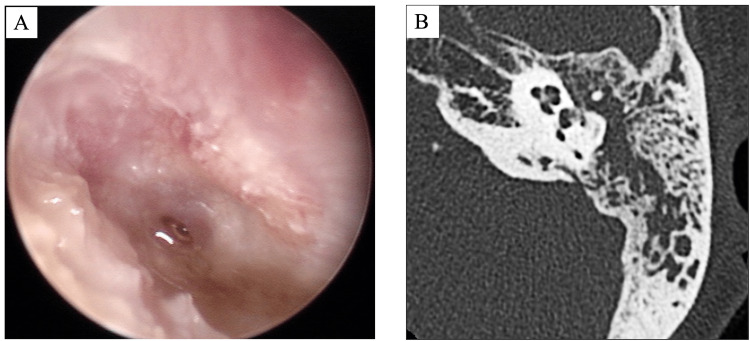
Images of the otitis media and mastoiditis prior to treatment (A) Flexible endoscopy showing perforated left tympanic membrane with otorrhea. (B) Computed tomography scan of the left temporal bone showing soft-tissue density in the tympanic cavity and mastoid.
